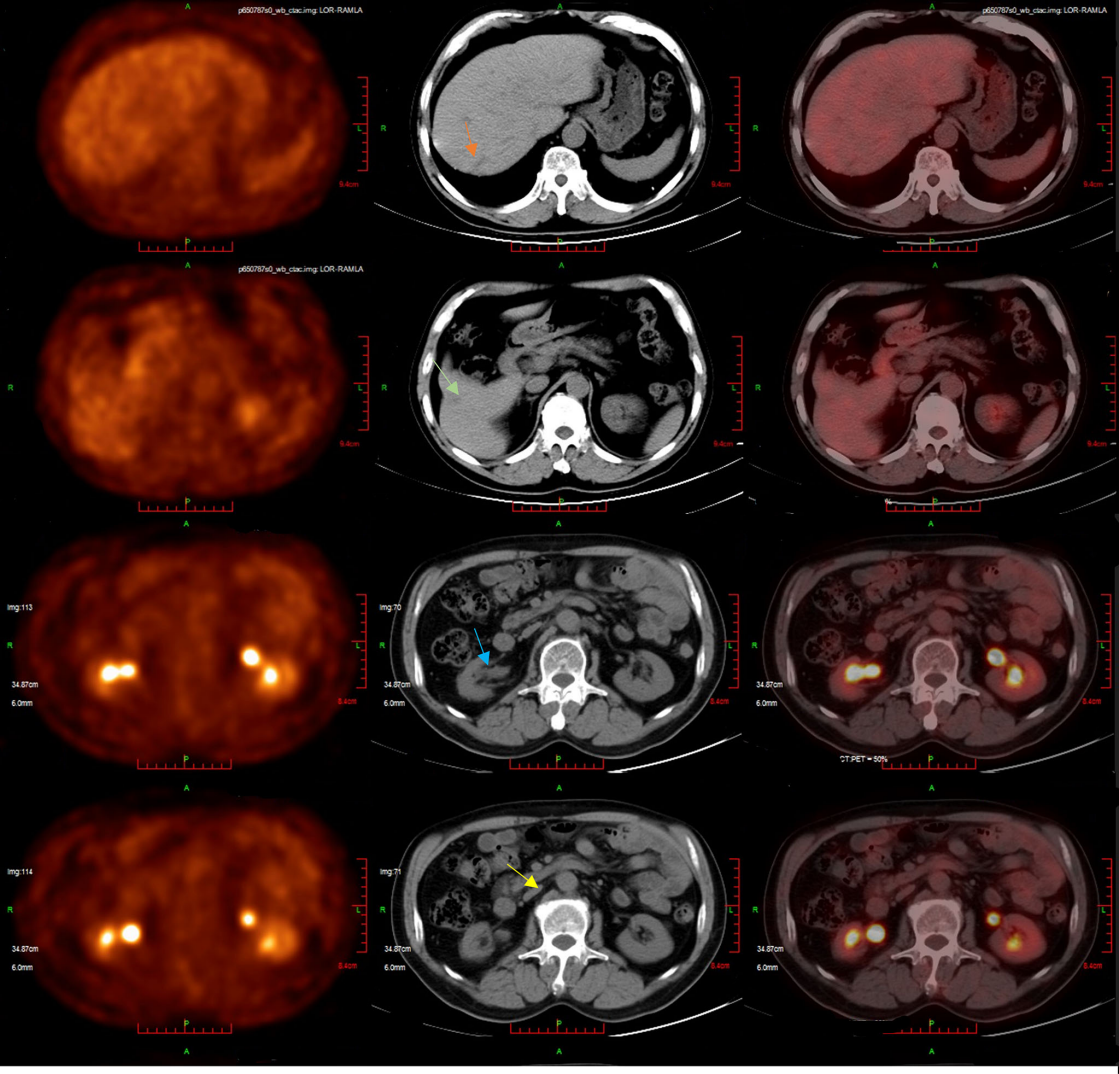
The patient’s latest PET/CT showed a complete response on 25 August 2022. Several slightly hypodense shadows were seen in the liver parenchyma, none of which showed abnormally high ^18^F-FDG uptake. No definite signs of residual tumors were seen throughout the body.
